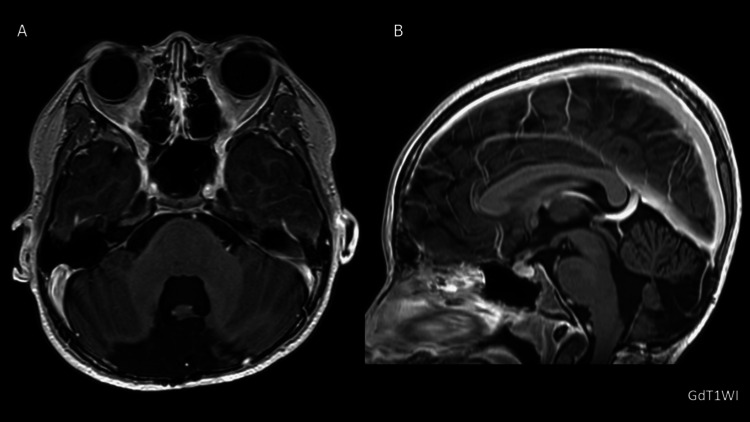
Long-term follow-up MRI after chemotherapy and stem cell transplantation. (A, B) A follow-up gadolinium-enhanced T1-weighted MRI obtained more than 10 years after treatment revealed no evidence of medulloblastoma recurrence.
